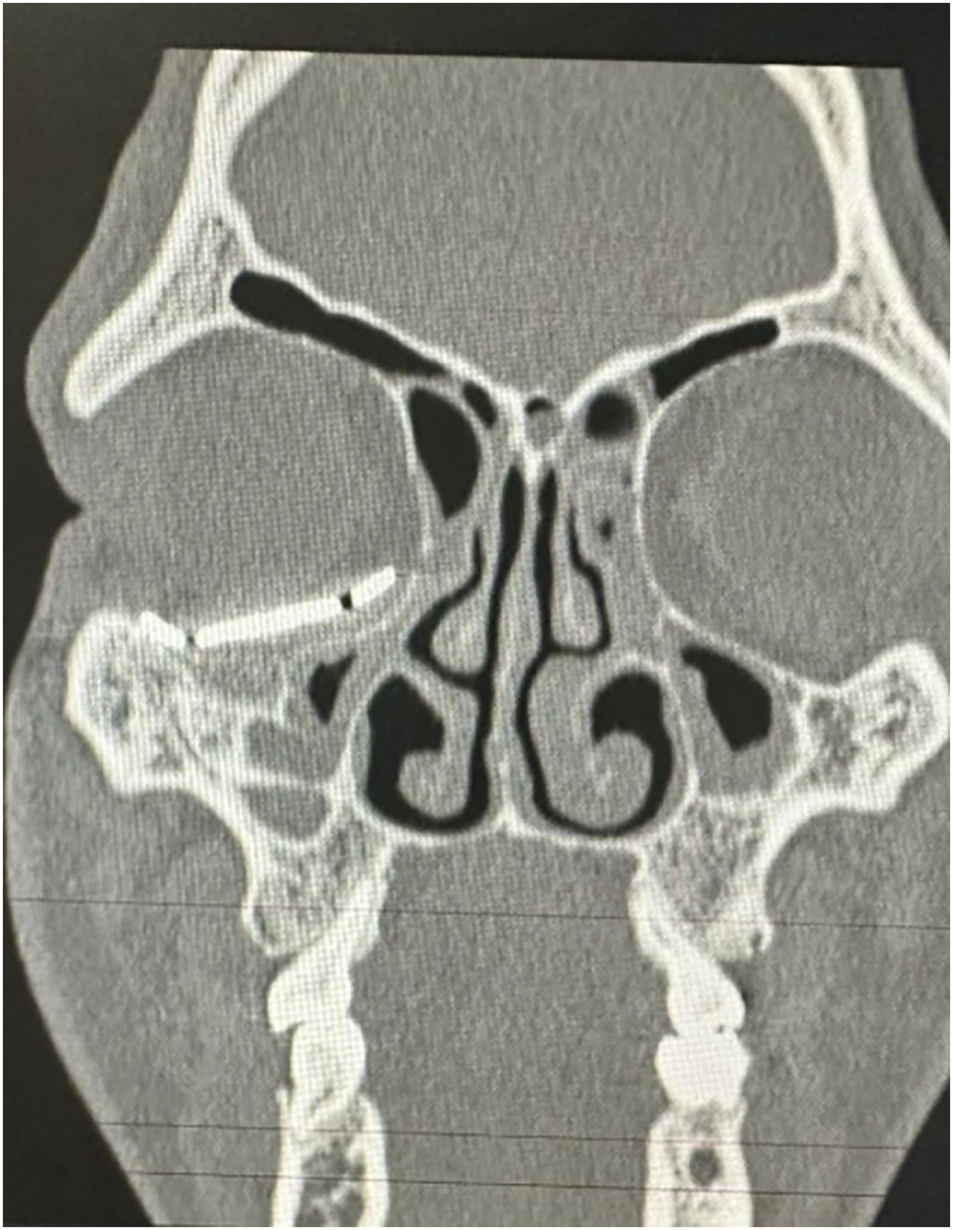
Coronal CT scan of the face showing the adaptation of the customized prosthesis.
